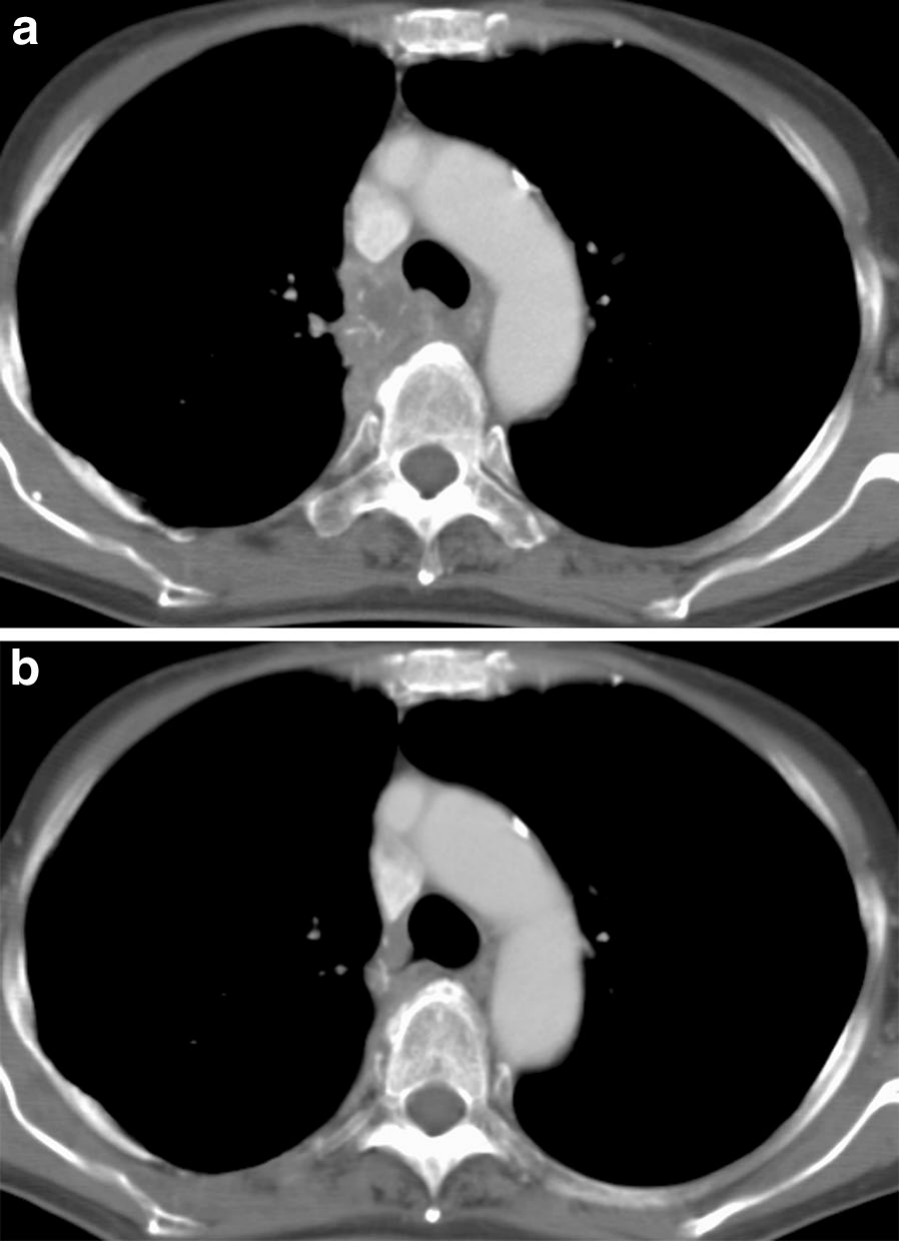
Chest computed tomography images of mediastinal lymph nodes (#4R) before (a) and 6 months after gefitinib treatment (b)
